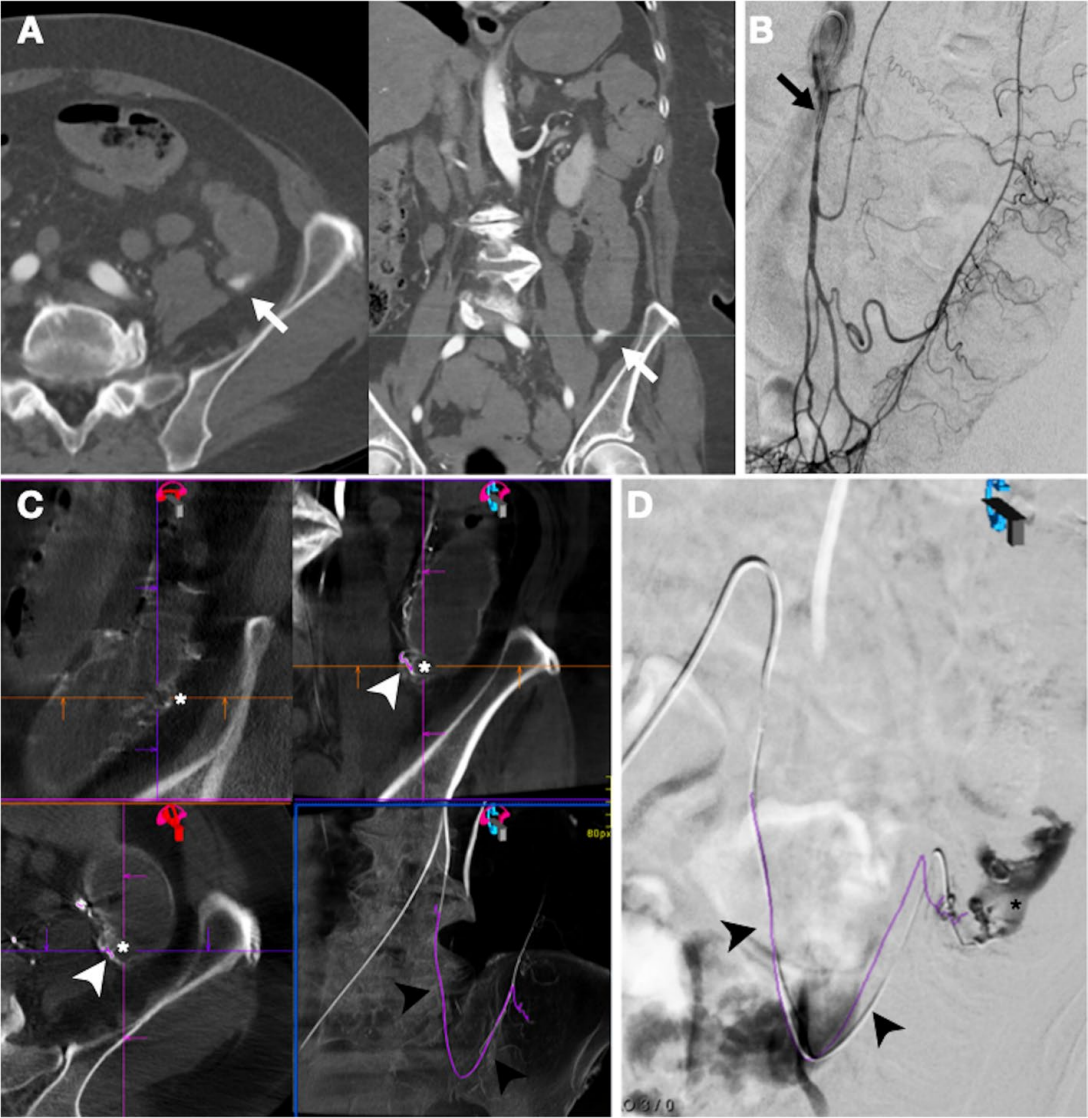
Empiric TAE approach in patient 1 A Pre-procedural arterial-phase CTA MPR images demonstrates an extravasation in sigmoid diverticula (white arrow). B No extravasation on subsequent DSA with contrast injection in the inferior mesenteric artery (black arrow). C Volumetric inferior mesenteric CBCT dataset displayed as MPR images in the 4D workplace. Although again no bleeding could be demonstrated, this allowed to identify the culprit diverticula (white asterisk) based on prior CTA. Then, the adjacent vas rectum (white arrowhead), presumably responsible for diverticular arterial supply and LGIB, was pointed as target for vessel detection. After also designating the catheter tip as proximal starting point, the vessel trajectory to the defined target could be identified semi-automatically (black arrowhead). D 3D roadmap (black arrowhead) virtually displayed on fluoroscopy for real-time guidance. After engaging the targeted vas rectum with a microcatheter, super-selective contrast injection ultimately reveals an extravasation (black asterisk)
